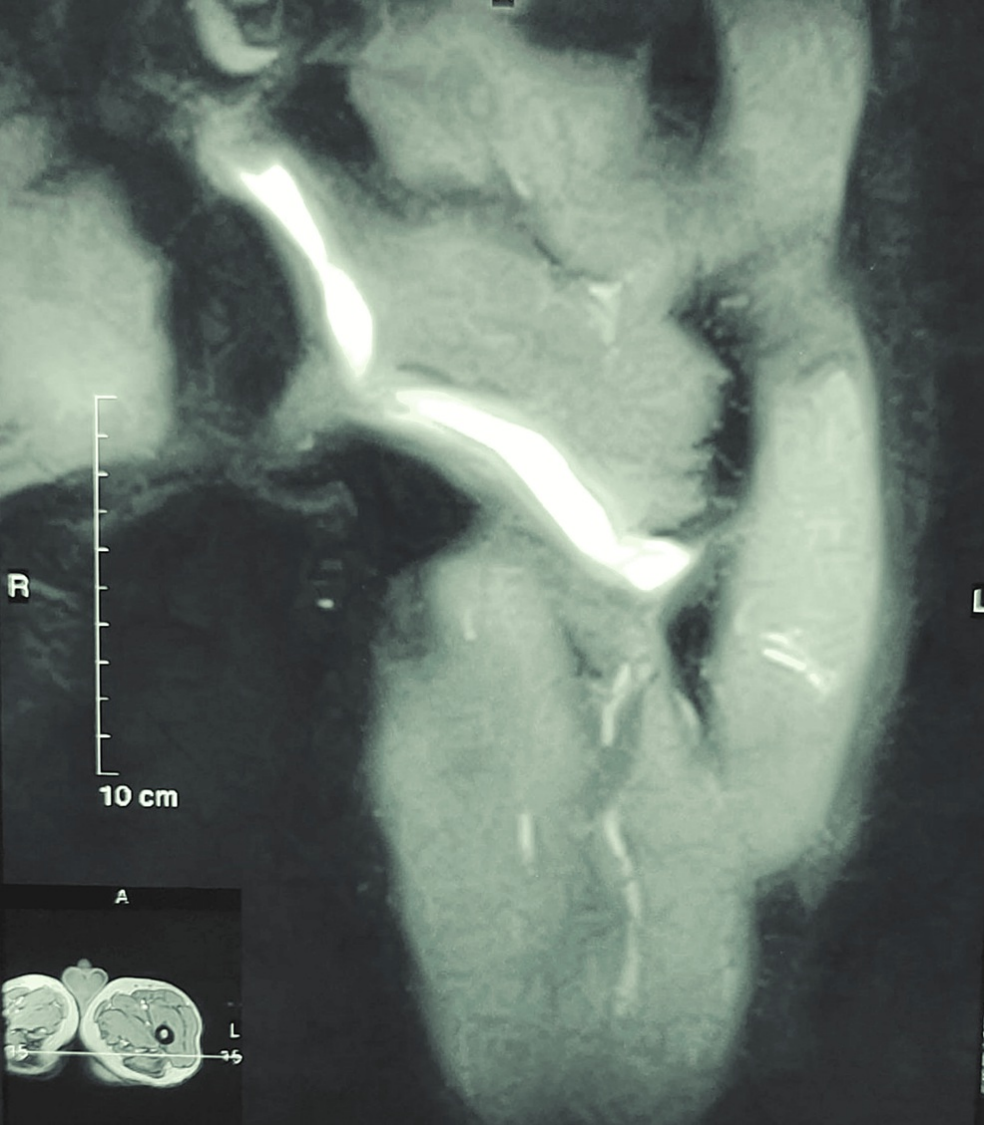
MRI of the left thigh with contrast showing a contrast-filled tract ascending from the skin of the posterior left thigh and ascending to bilateral ischioanal fossae
